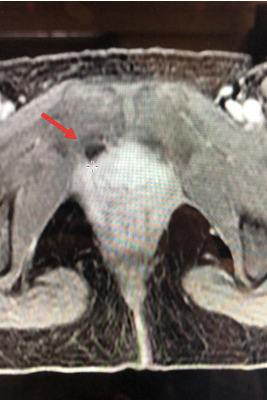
Cross section of the pelvic MRI, showing a peritoneal sac containing fat tissue herniating through the pelvic floor (red arrow).
